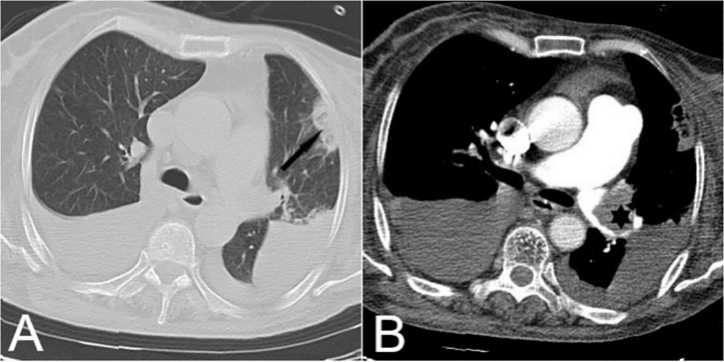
A) Axial lung window CT image demonstrated a patch of subpleural ground-glass change with a rim of consolidation, compatible with the reversed-halo sign. B) Axial mediastinal window, reveals a large filling defect (asterisk) in the left pulmonary artery with secondary pulmonary infarction.
